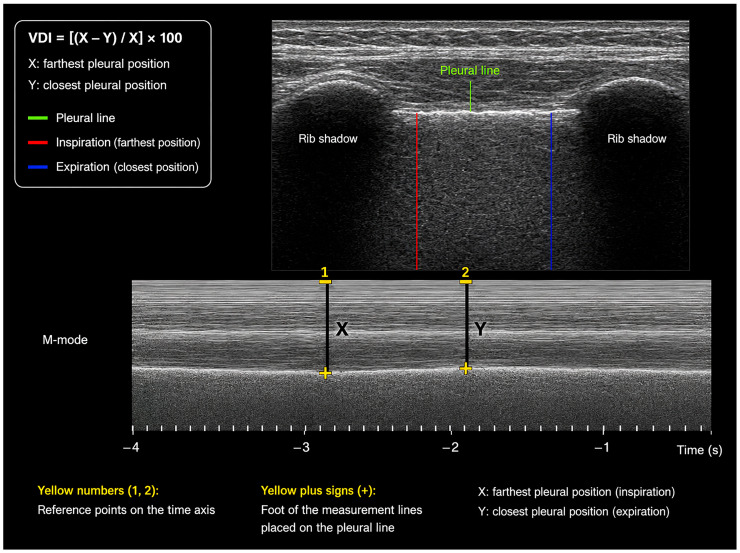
Measurement of the vertical displacement index (VDI) using M-mode lung ultrasound. VDI was calculated as the difference between pleural displacement during inspiration and expiration. The pleural line is shown with a characteristic seashore sign, and displacement was quantified between the farthest (X) and closest (Y) pleural positions over time.
